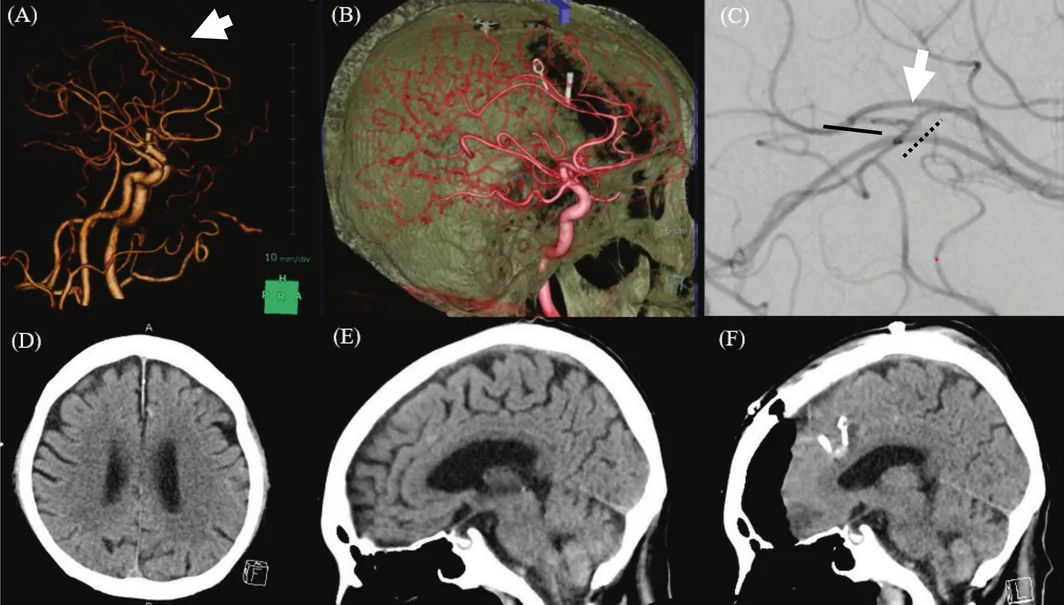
Neuroimaging findings of the cerebral mycotic aneurysm. (A) Contrast‐enhanced computed tomography angiography before craniotomy demonstrating a saccular aneurysm measuring approximately 4 mm in the left anterior cerebral artery (arrow). (B, C) Cerebral angiography after trapping and bypass surgery demonstrating a patent end‐to‐side anastomosis between the right pericallosal artery and the left callosomarginal artery. In panel C, the right pericallosal artery (solid line), left callosomarginal artery (dashed line), and anastomotic site (arrow) are indicated. (D, E) Preoperative non‐contrast computed tomography demonstrating a high‐density area suggestive of subarachnoid hemorrhage adjacent to the aneurysm. (F) Postoperative computed tomography after trapping and bypass surgery showing no progression of intracranial hemorrhage.
